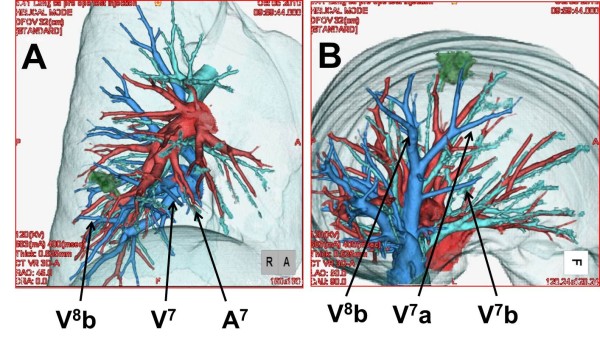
Images from three-dimensional computed tomographic angiography of the right lower lobe of the lung. The complicated anatomy of the pulmonary arteries (red), pulmonary veins (blue), and bronchus (green) is precisely depicted. From this image, the intersegmental plane between medial basal segment (S7) and anterior basal segment (S8) can be easily imagined.
